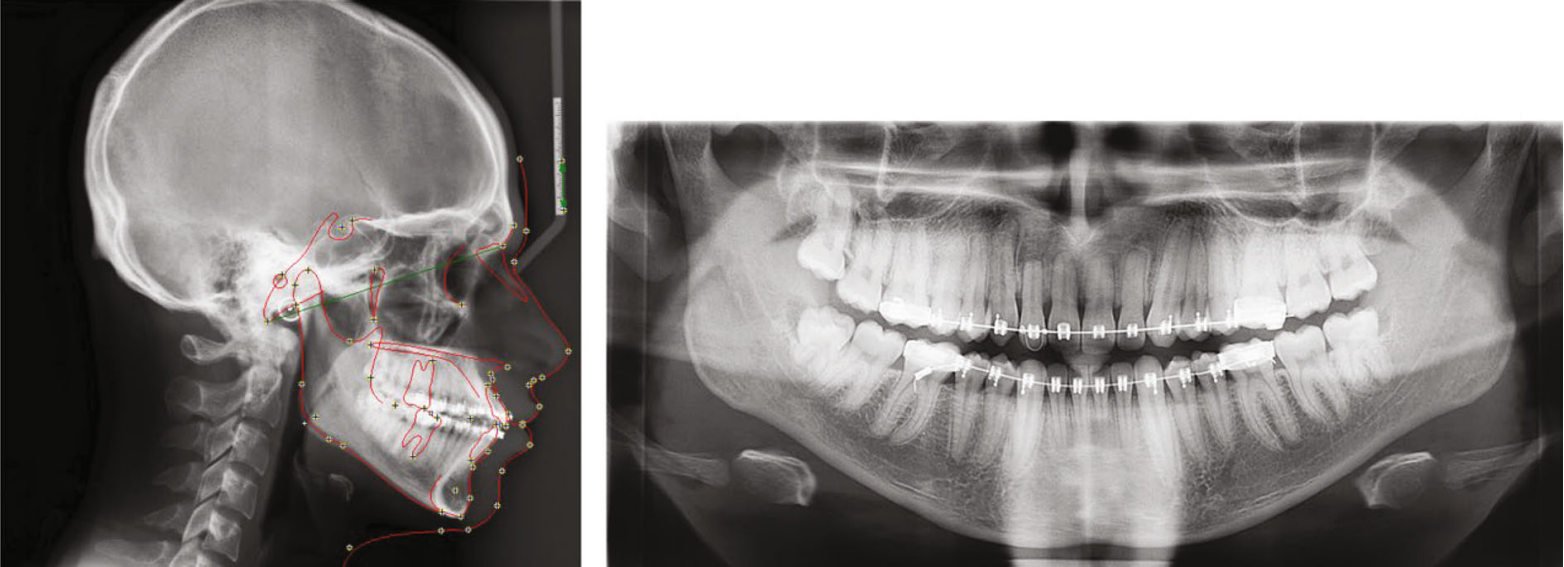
Post-treatment lateral cephalogram with tracing and orthopantomogram.
